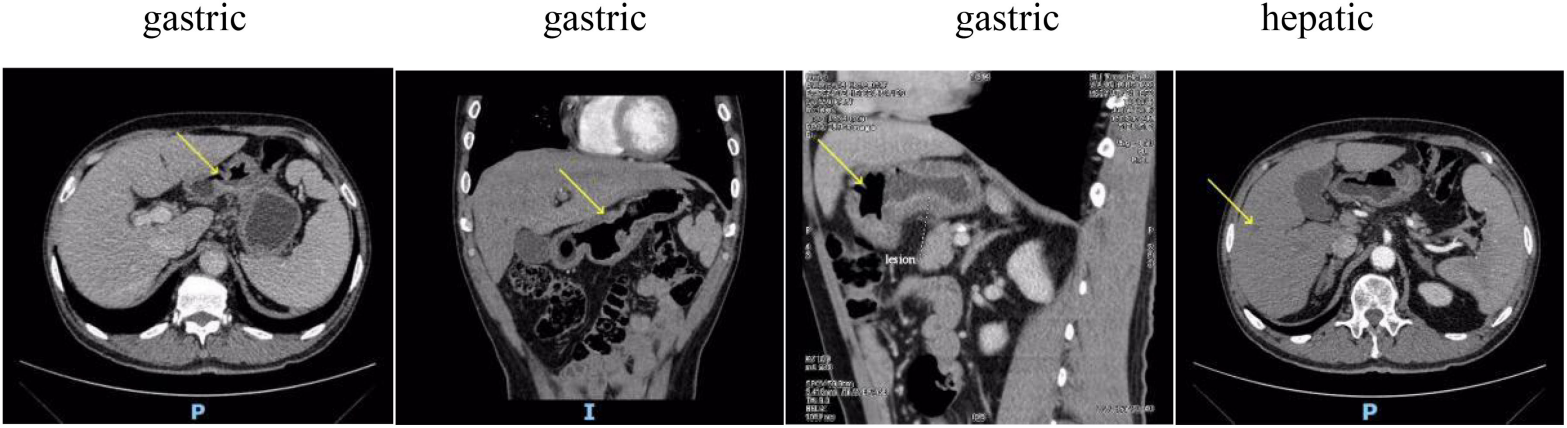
June 2015, CT after six cycles of Oxaliplatin combined with S-1 chemotherapy. Yellow arrows indicate the location of the lesion.
